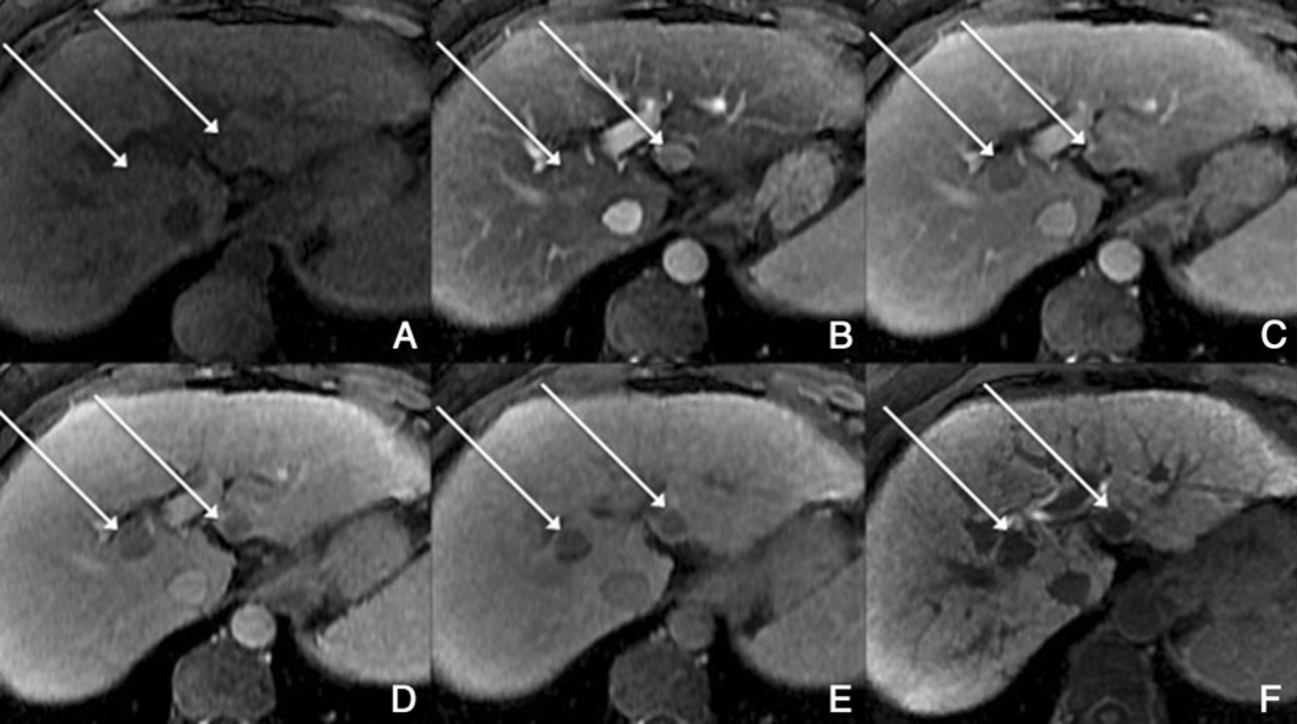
Hypervascular HCCs in a 50-year-old man with chronic liver disease. a Pre-contrast MRI, b arterial phase, c portal vein pressure (PVP), d 3 min, f 4 min, f HCP. Marked heterogeneous liver with nodular contour, with reticulation in the HCP- cirrhotic The *arrows* point to two rounded focal lesions, with arterial enhancement (one less prominent), PV washout and hypointensity in the HCP
